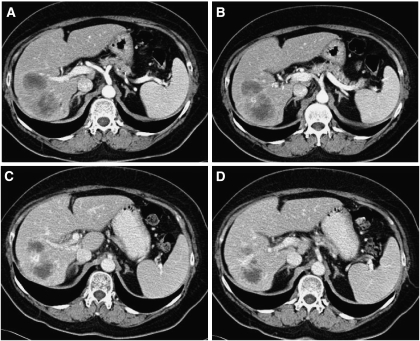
Contrast-enhanced CT scans of patient in Figure 1, showing pre-radioembolisation hypoattenuating lesions (A/B); 3-month post-radioembolisation decreased lesion size, decreased intra-lesional vascular enhancement, increased intra-lesional necrosis, thin peripheral enhancement (C/D) and reduced compression/narrowing of large posterior right portal vein branch (D).
